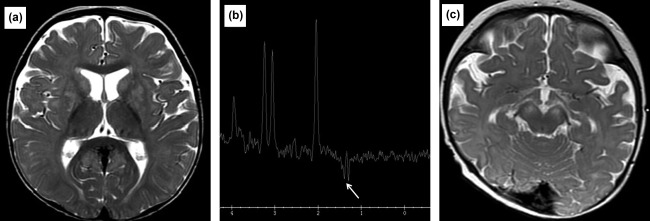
(a) MRI image of Patient 2 at 9 months of age. Axial T2W image at the basal ganglia level demonstrating symmetrical hyperintense foci involving the lentiform nuclei and caudate heads. The thalami are spared. (b) MRS of Patient 2 at 9 months of age. Long TE MRS with left basal ganglia sampling demonstrating a lactate peak at 1.3 ppm. (c) MRI image of Patient 3 at 8 months of age. Axial T2W image at midbrain level, demonstrating bilateral symmetrical hyperintensity of the cerebral crura and subtle hyperintensity of the periaqueductal gray matter
